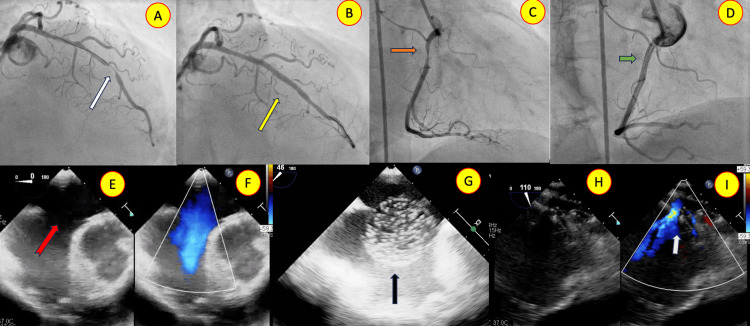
(A) Left coronary angiogram showing tight obstruction of the left anterior descending artery (white arrow), (B) post-stenting good flow across the narrow segment is seen (yellow arrow). (C) Right coronary artery angiogram showing tight narrowing (orange arrow), (D) good post-stenting flow is visualized (green arrow). (E, F) Transesophageal echocardiogram (TEE) with color Doppler showing the secundum atrial septal defect (red arrow). (G) Balloon (black arrow) occlusion was done to demonstrate the left ventricular diastolic pressure change. (H, I) TEE with color Doppler post-fenestrated device closure showing the stable device position and fenestration flowing left to right (light green arrow).
